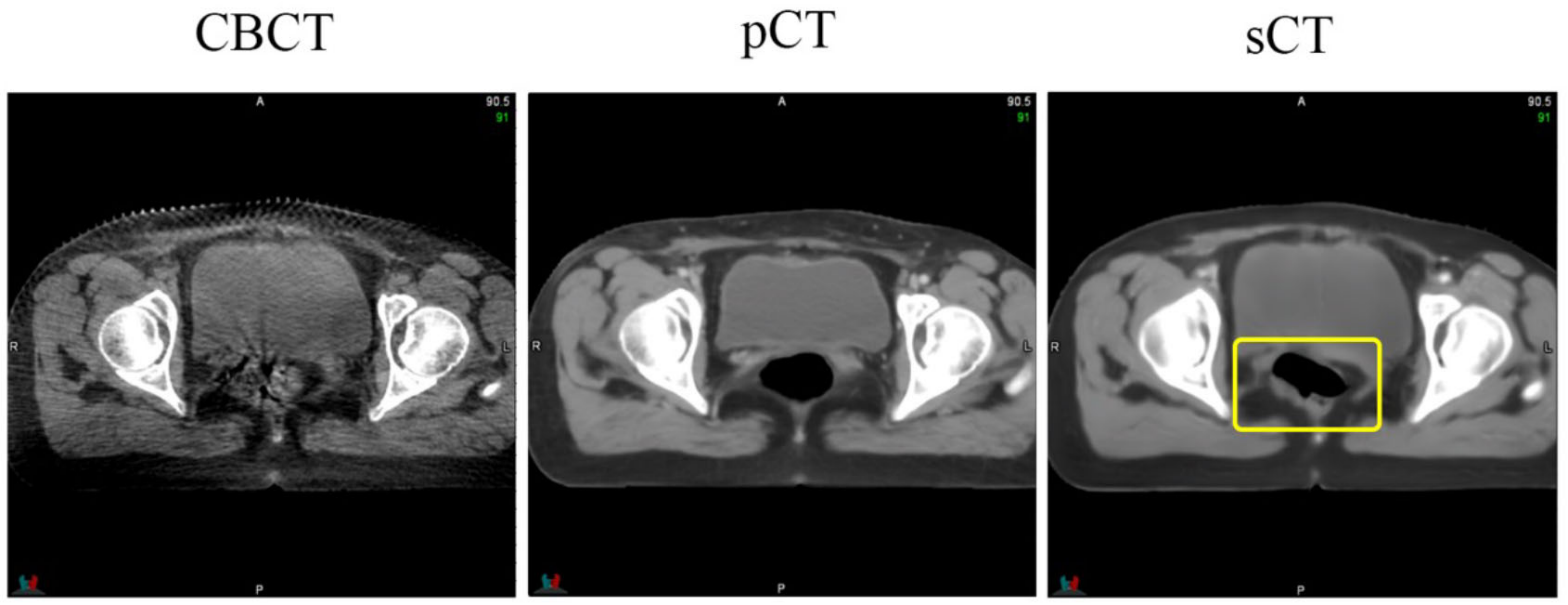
An instance of hallucinations on an sCT image. The area highlighted by the yellow box indicates the location of the hallucinations, which are not present in the CBCT image.
